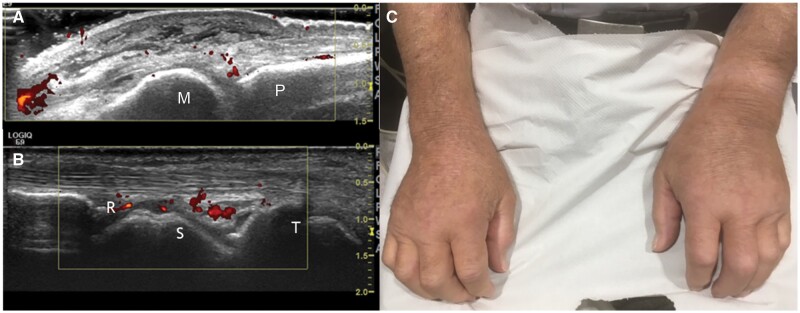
(A) Longitudinal dorsal view of a metacarpophalangeal joint. Presence of mild intra-articular synovitis and widespread non-specific extracapsular subcutaneous oedema. M, metacarpal; P, phalanx. (B) Longitudinal dorsal view through the radioscaphoid aspect of the wrist on ultrasonography. Presence of mild low-level synovitis with increase power Doppler signal. R, radius; S, scaphoid; T, trapezium. (C) R’s hands. Swelling over the dorsum aspect of the hands with marked pitting oedema upon palpation
